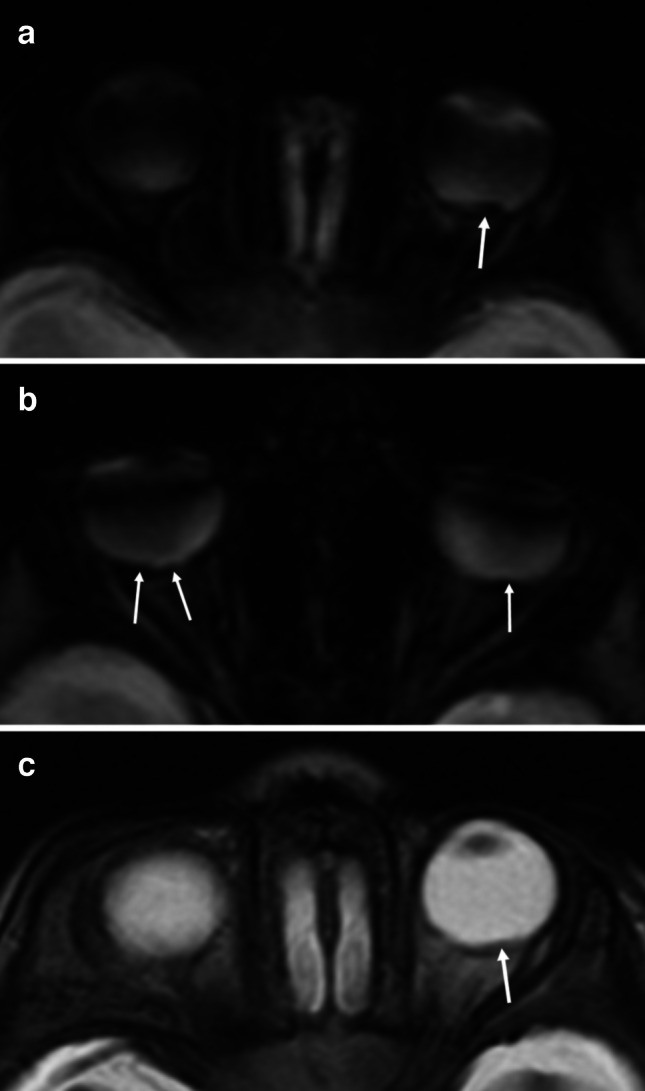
5-month-old girl with abusive head trauma following shaking and without retinal hemorrhages on fundoscopy. a, b Axial T2*-weighted magnetic resonance images through different orbital planes show subtle signal changes along the retina consistent with bilateral retinal hemorrhages (*arrows*). c Axial T2-weighted magnetic resonance image through the orbits demonstrates an abnormality at the dorsal pole of the left retina compatible with retinal hemorrhages (*arrows*)
